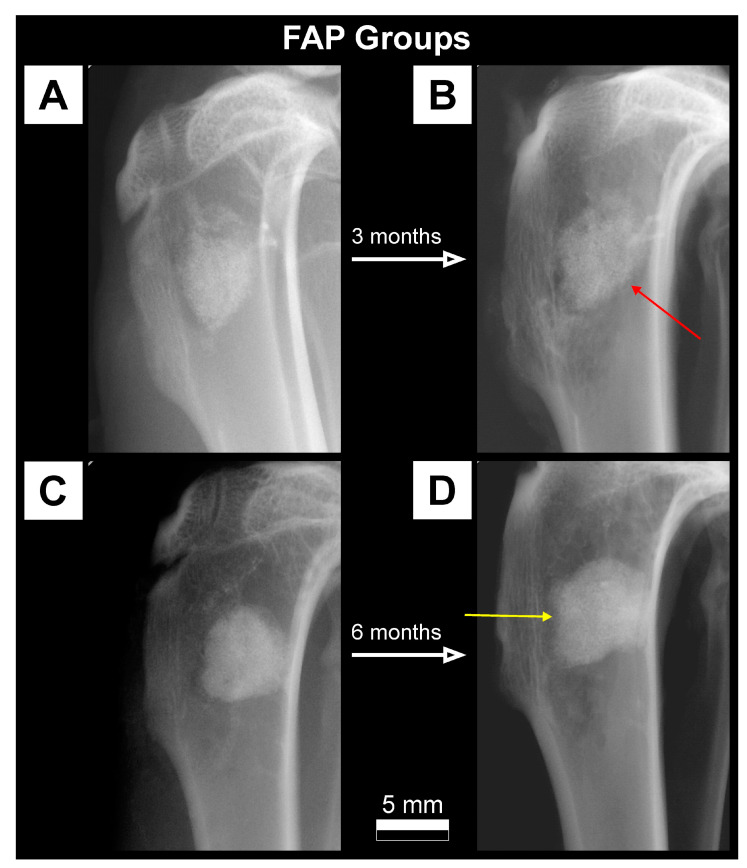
Lateral radiographs of a defect in the proximal tibial metaphysis filled with FAP granules immediately after the procedure (A) and after 3 months (B) as well as immediately after the procedure (C) and after 6 months (D). After three months of healing, (B) a stripe of low density shadow is visible on the edge of the filling (red arrow). After six months, the stripe of low density shadow is less visible. There are periosteal reactions (yellow arrow) on the margins of the filling and greater assimilation of the FAP material with the surrounding bone.
